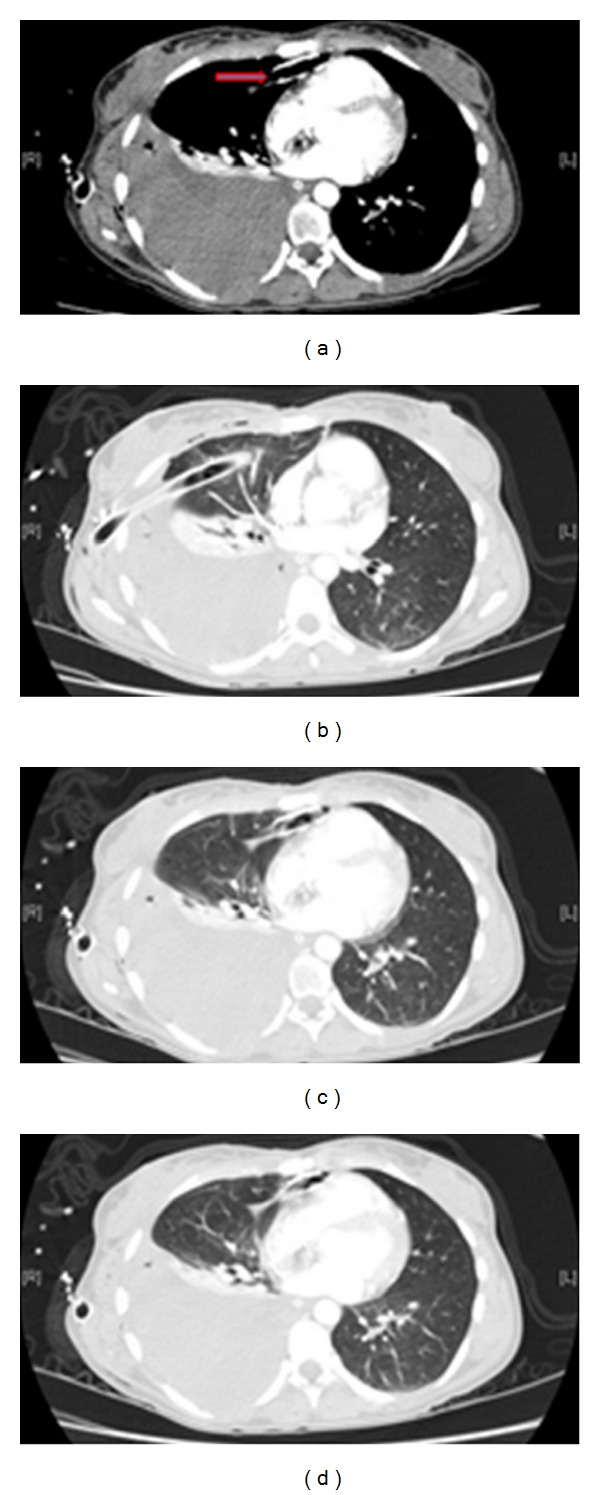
Axial CT scans on the same patient as in Figure 22 showing inappropriately placed chest drain in an attempt to drain a right basal pleural effusion.
